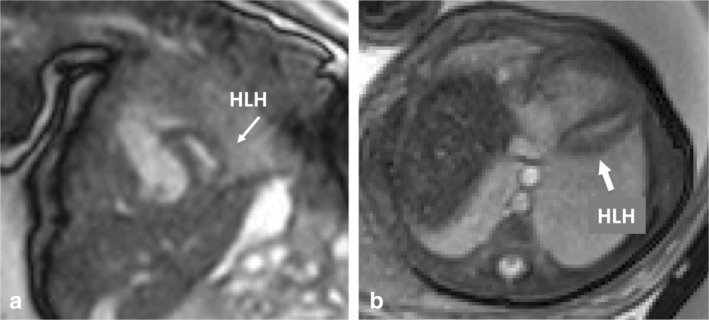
Two examples of fetal 3.0 T balanced steady‐state free precession (b‐SSFP) cine images acquired with Doppler ultrasound (DUS) gating. (a) Fetus GA 35 + 3 with hypoplastic left heart (HLH) showing paradoxical septal motion on short axis views (Movie S3a in the Supplemental Material). (b) HLH example in axial view (Movie S3b in the Supplemental Material).
